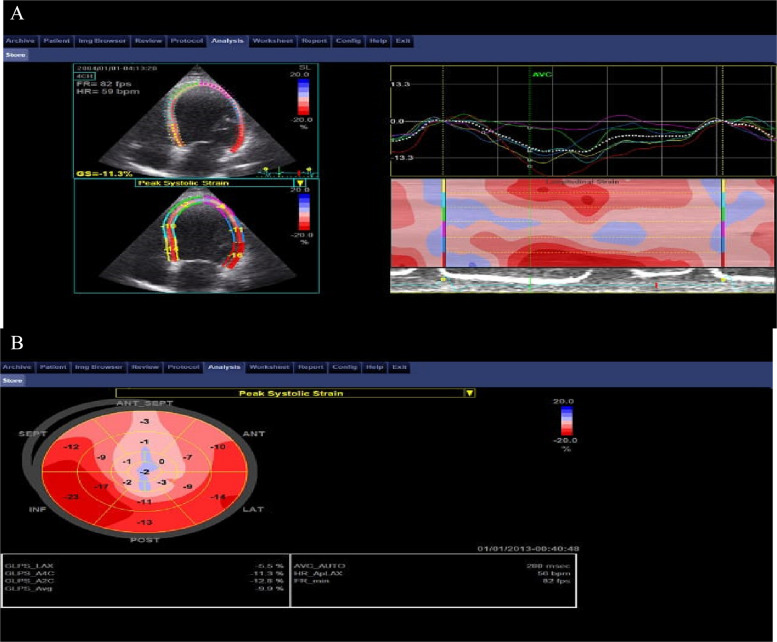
Curves (A) and Bull’s eye (B) displays of the segmental longitudinal strain measured by 2D speckle-tracking echocardiography in apical views
